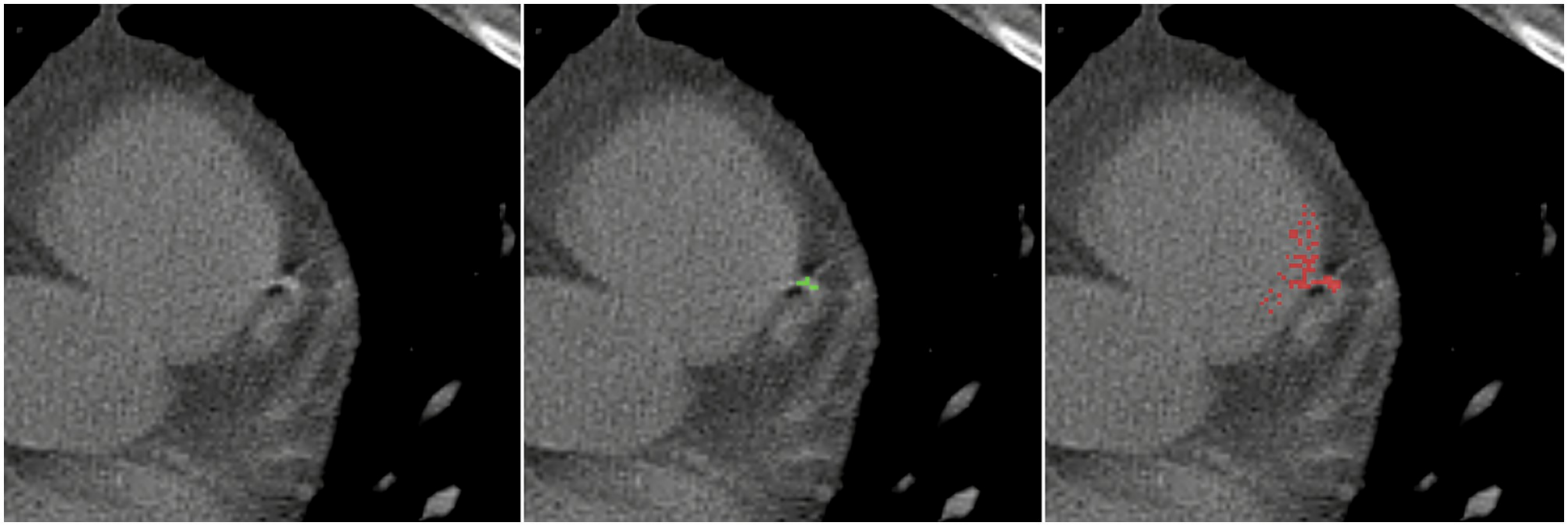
Left: Cropped CT slice containing CAC lesion; Middle: Segmented CAC lesion ≥ 130 HU (green); Right: Segmented region using ≥ 90 HU around the CAC lesion (red).
